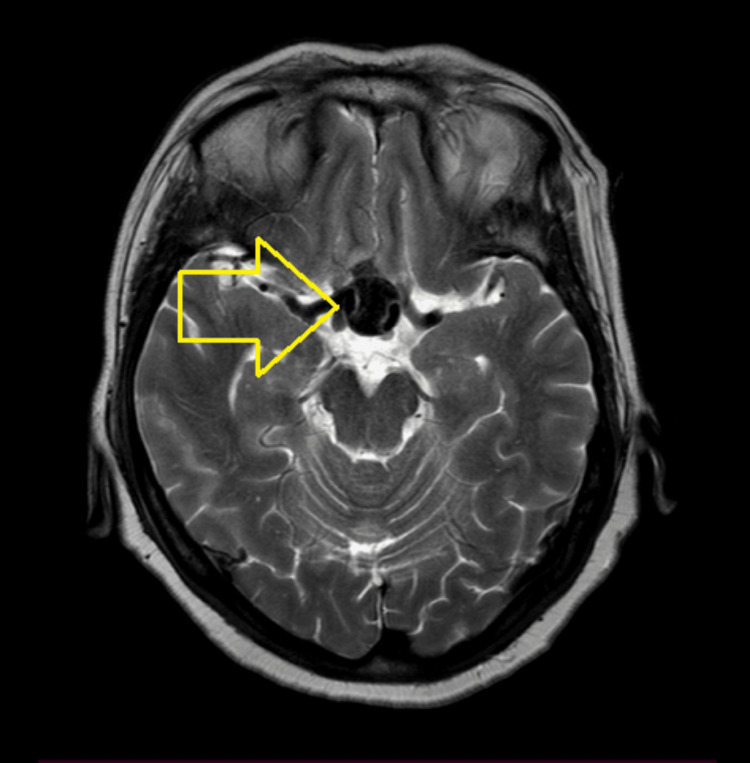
Axial T2-weighted MRI showing a well-defined suprasellar lesion demonstrating marked hypointensity, in close proximity to the internal carotid arteries (arrow). MRI: magnetic resonance imaging
